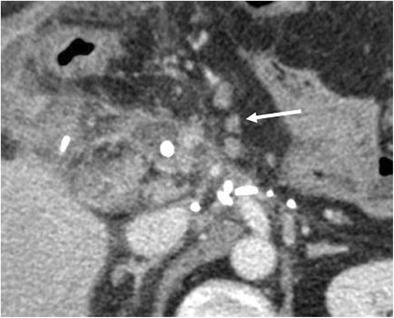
Inflammatory adenopathy. Axial CT image shows the presence of enlarged lymph nodes (white arrow) in the mesentery, close to the surgical bed
